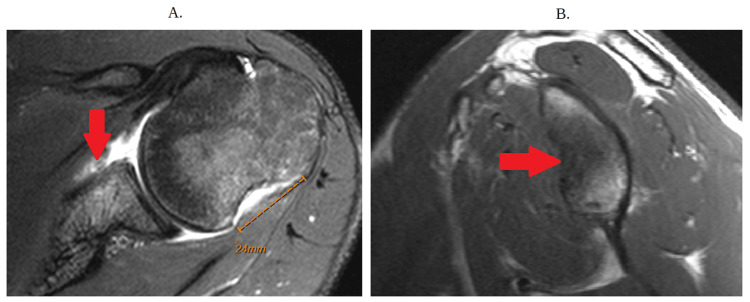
Magnetic resonance imaging without contrast (a) The arrow depicts the labral tear. A large Hill-Sachs lesion of approximately 24 mm indicating evidence of failed remplissage is also noted. (b) The red arrow depicts bone loss
